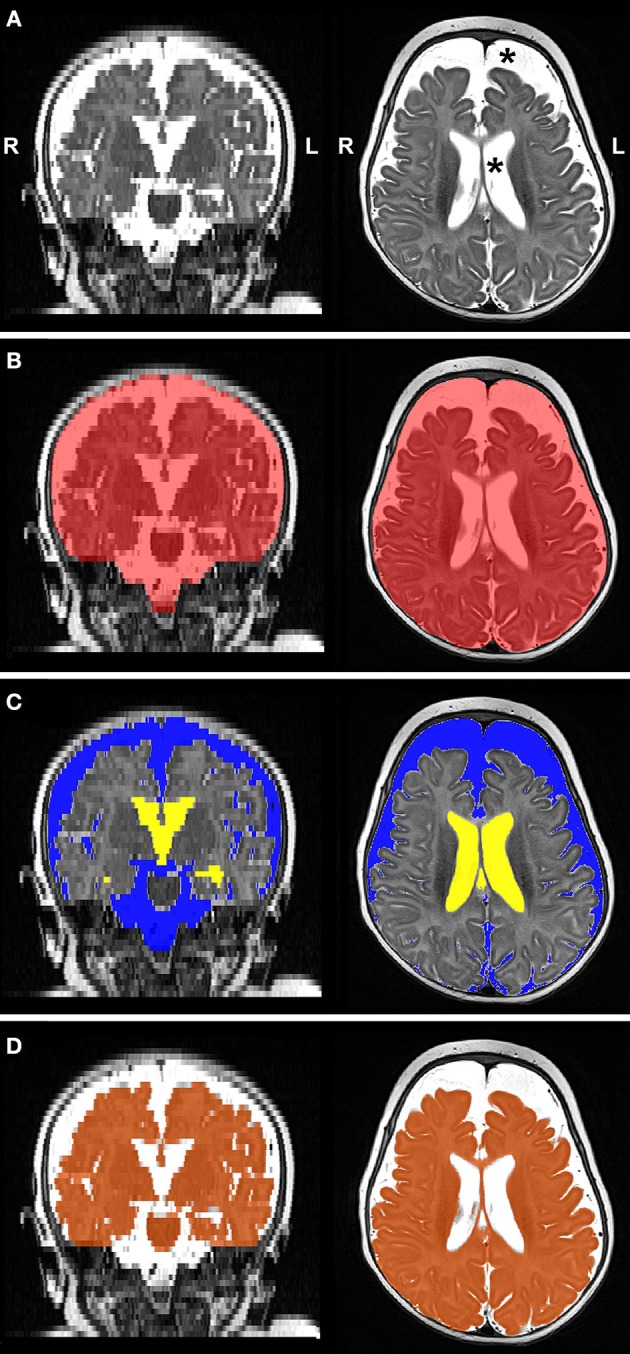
Representative T2-weighted image (A; gray-scale) and its final edited MANTiS segmentations of intracranial space (B; red), total CSF subdivided into extra-axial space (C; blue) and ventricular system (C; yellow), and whole brain (D; orange) in a full-term patient scanned at 3.4 months of age. Note increased CSF volume (asterisks in Panel A). L, left; R, right.
